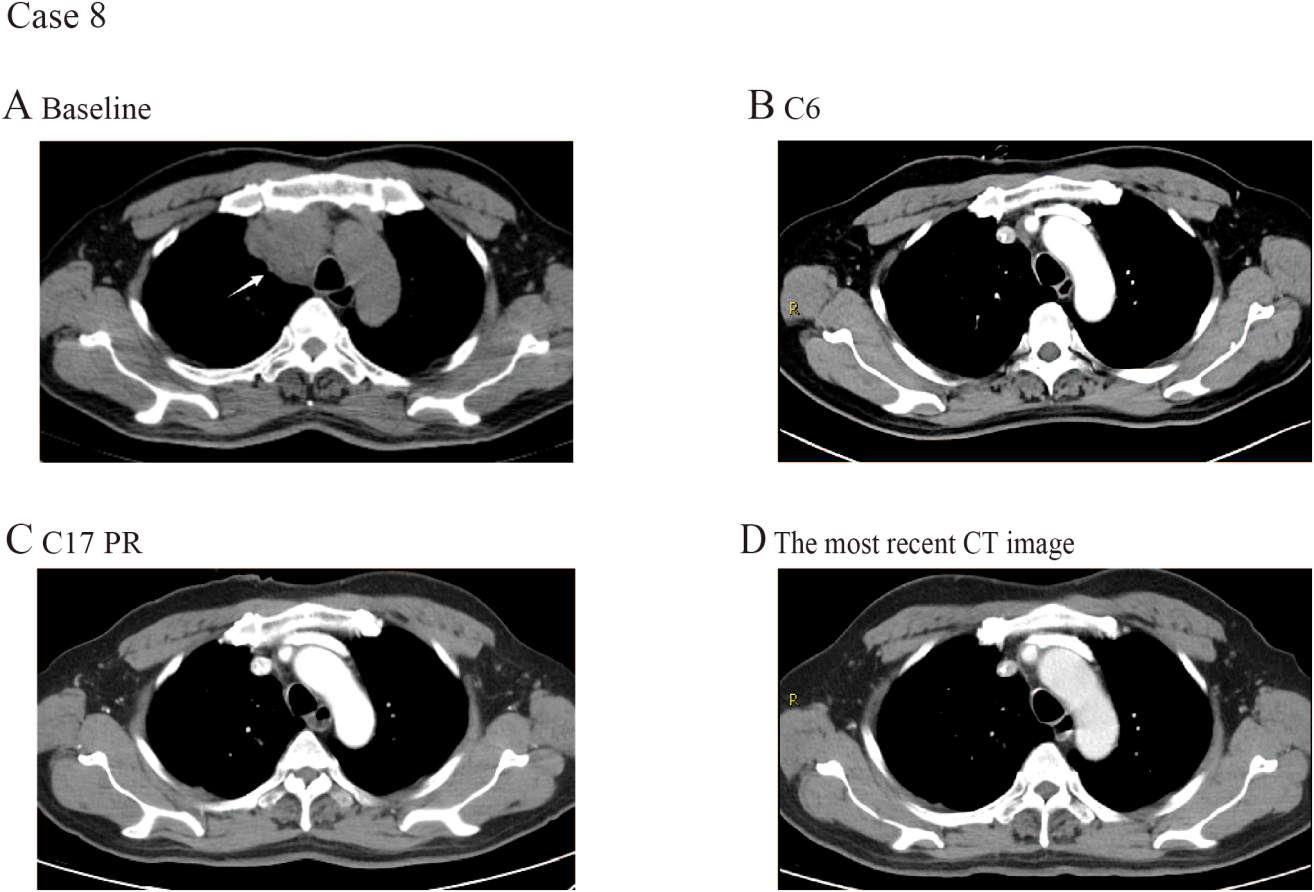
CT images selected from case 8. (A) CT scan examination of the primary tumor at the first time of diagnosis. (B) The primary tumor size decreased after 6 cycles of treatment. (C) The primary tumor size decreased after 17 cycles of treatment. (D) The most recent CT image.
